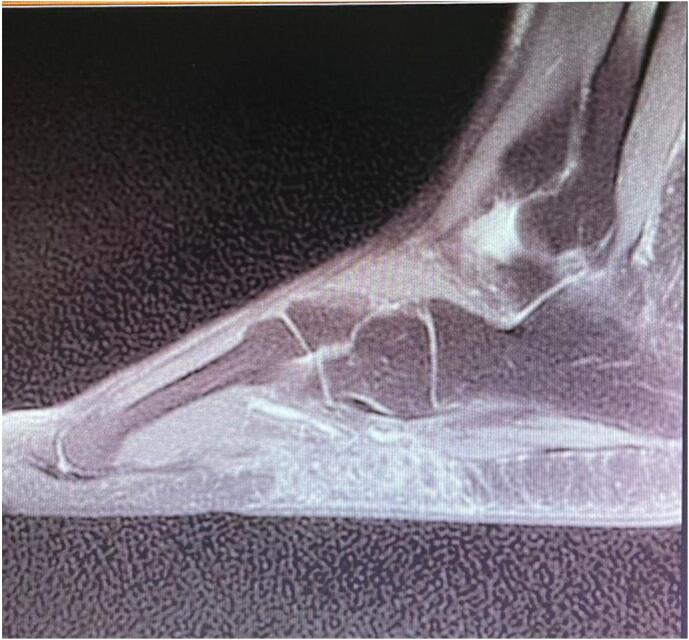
Magnetic resonance imaging showing an extensive inflammatory edematous infiltrate affecting the lateral edge of the foot and the sole of the foot, of dermo-hypodermal location and extending to contact with the plantar fascia without any real visible collection or bone or osteoarticular extension.
